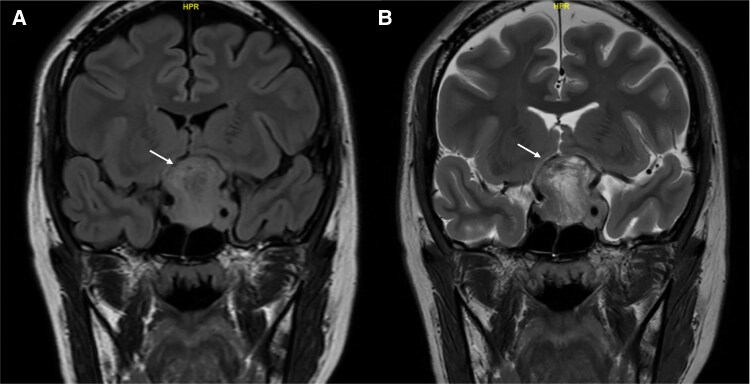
(A) Coronal section of unenhanced T2-weighted FLAIR MRI images of the brain preoperative. (B) Coronal section of unenhanced T2-weighted MRI images of the brain preoperative. Abbreviations: FLAIR, fluid-attenuated inversion recovery; MRI, magnetic resonance imaging.
